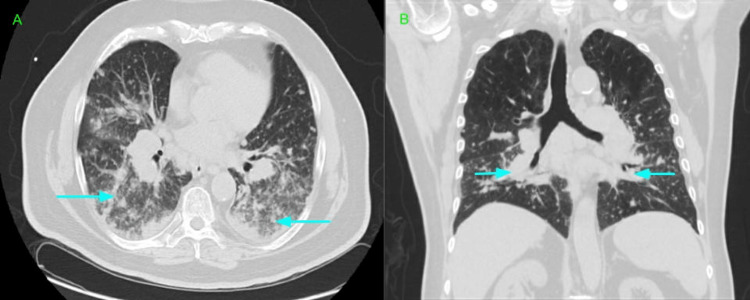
Chest CT without contrast (A: axial view; B: coronal view) revealed innumerable confluent nodules in both lungs with basilar predominance. There were ground-glass opacities in the lungs affected by the nodules (blue arrows).
